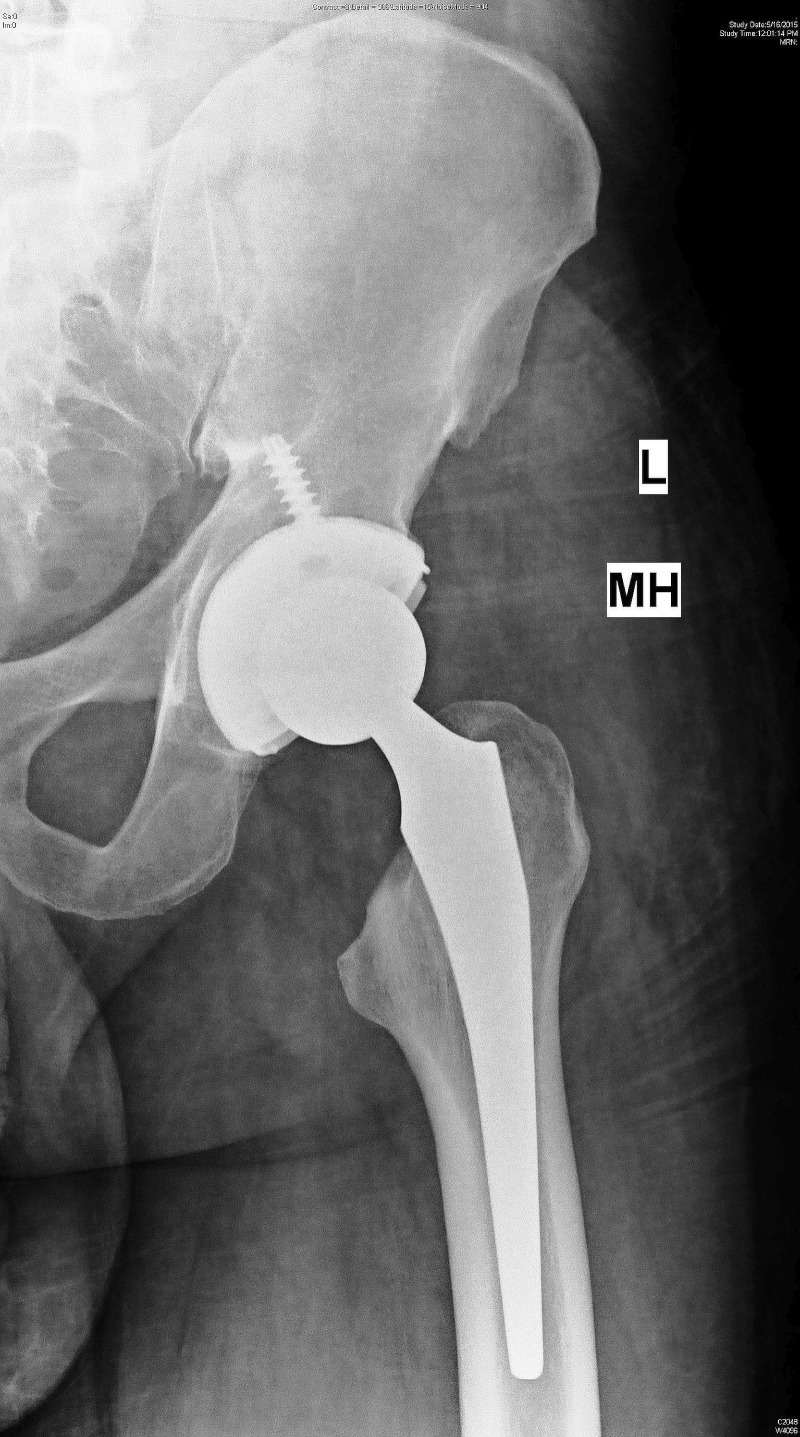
Postoperative radiograph (anteroposterior view) of the left hip showing total hip replacement.
